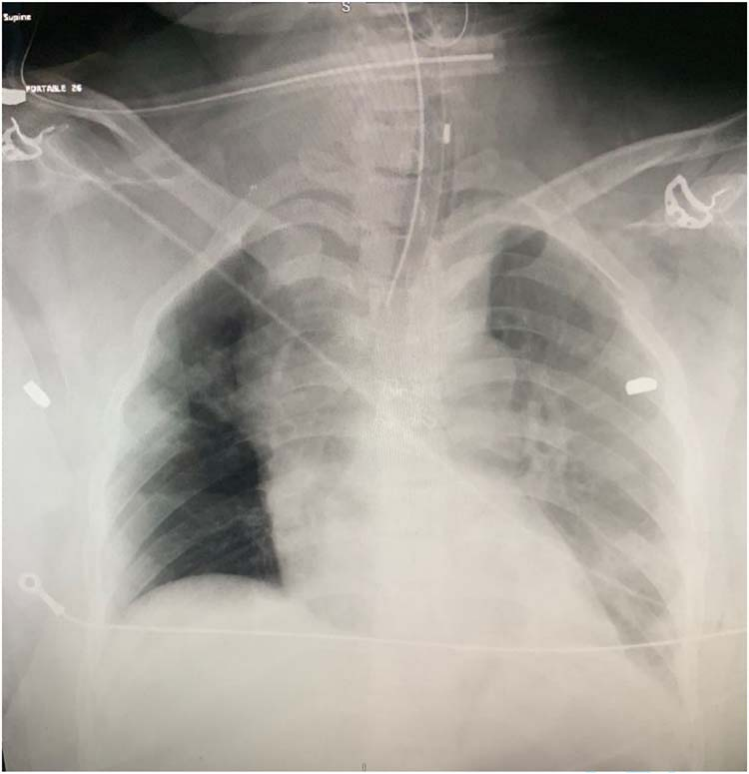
Chest radiograph showing bullet fragments in the chest cavity.
